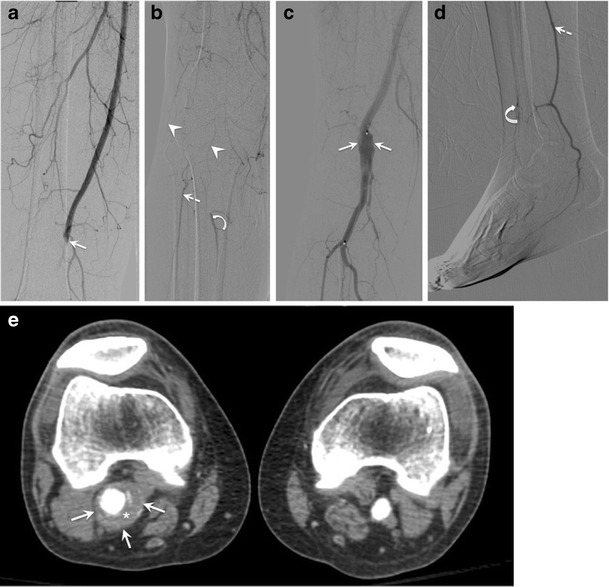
Popliteal artery aneurysm (PAA) in a 52-year-old man admitted for cold right foot. (a, b) Digital subtraction angiography on admission shows abrupt occlusion of the proximal popliteal artery at the level of the adductor hiatus (*solid arrow*), with minimal collateral vessels around the knee (*arrowheads*), suggesting acute thrombosis. Distally, there is revascularization of the peroneal (*dashed arrow*) and anterior tibial (*curved arrow*) arteries. Patient underwent intravascular catheter-directed thrombolysis, and lytic check at 12 h (not shown) showed incomplete lysis of the thrombus. Patient was continued on the same treatment. (c, d) Digital subtraction angiography at 22 h post-lysis reveals a large PAA (*solid arrows*), with re-permeabilization of the posterior tibial artery (*dashed arrows*). However, the distal anterior tibial artery is barely opacified, suggesting vascular changes from chronic distal embolization resulting in tissue loss. (e) Axial CT angiography shows a larger PAA than expected from angiography images (*solid arrows*), with mural thrombus (*asterisk*). These findings characterize classical acute (acute thrombosis) and chronic (loss of distal run-off from repeated distal embolization) complications from PAA
